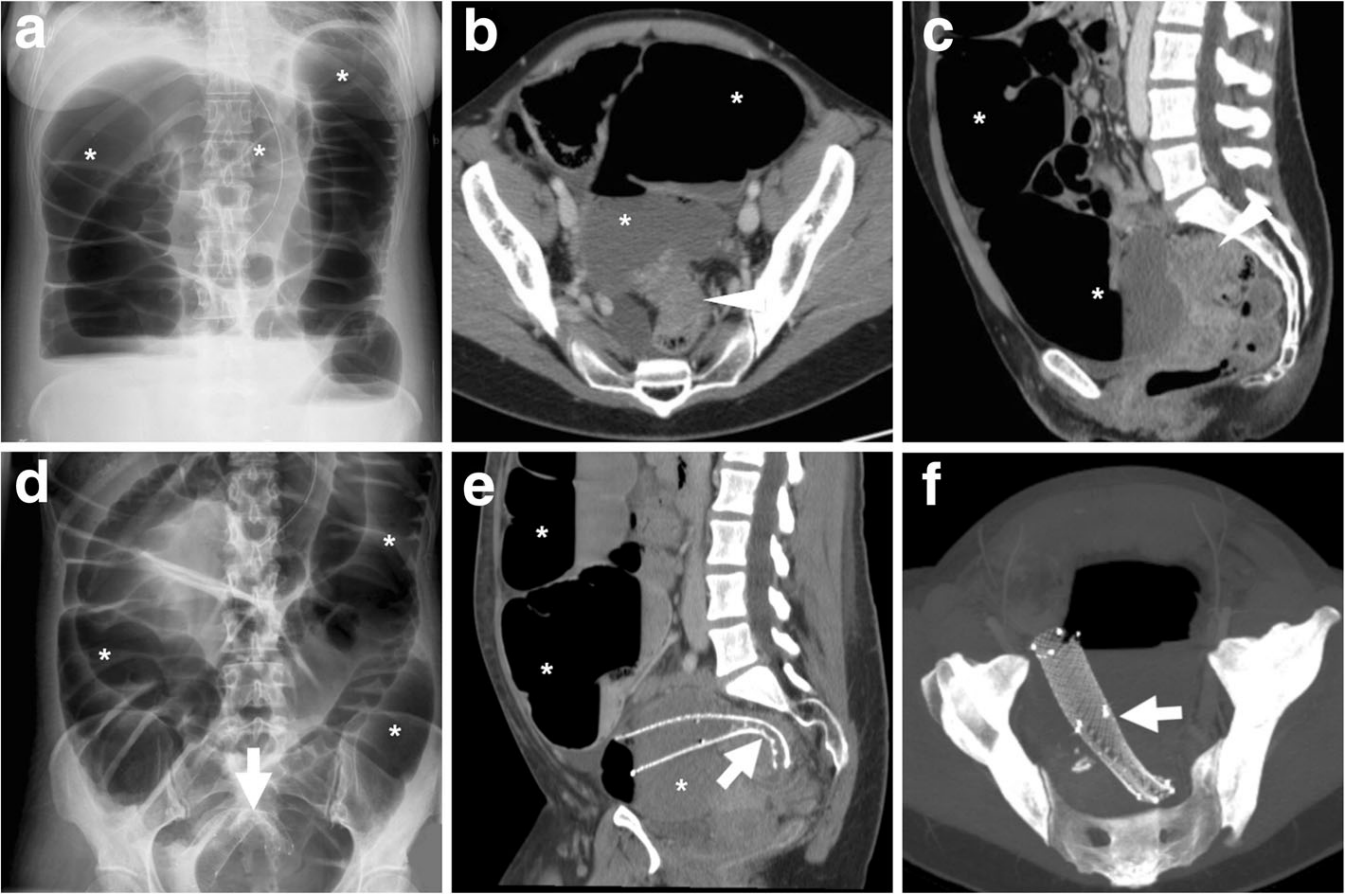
Failed relief of LBO from SEMS treatment of deep infiltrating endometriosis. Initial upright radiograph (a) showed severe colonic dilatation (*) with prominent air-fluid levels consistent with high-grade obstruction. Axial (b) and sagittal (c) CT images confirmed LBO (*) above a segmental mural thickening (arrowheads) at the rectosigmoid junction, which corresponded at pathology to endometriosis. After SEMS (thick arrows) positioning at an impassable extrinsic stricture 15 cm above the anal verge, radiographic follow-up (d) and CT (e, f) showed persistent LBO (*). Sagittal (e) and MIP (f) CT images showed incomplete expansion of the SEMS (thick arrows). Immediate laparotomy with rectosigmoid resection and temporary stoma was required
